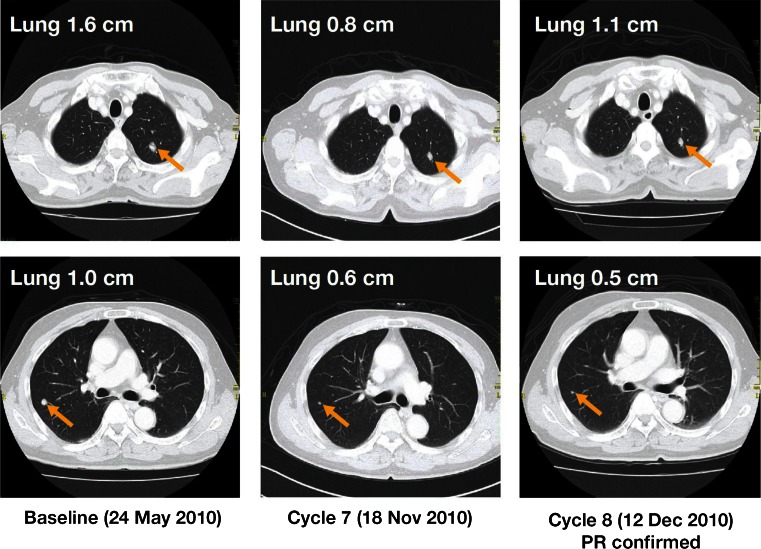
Computed tomography scans of lung metastases in a patient with a confirmed PR following treatment with AUY922 54 mg/m^2^ (63 years old, male, primary rectal carcinoid tumor)
